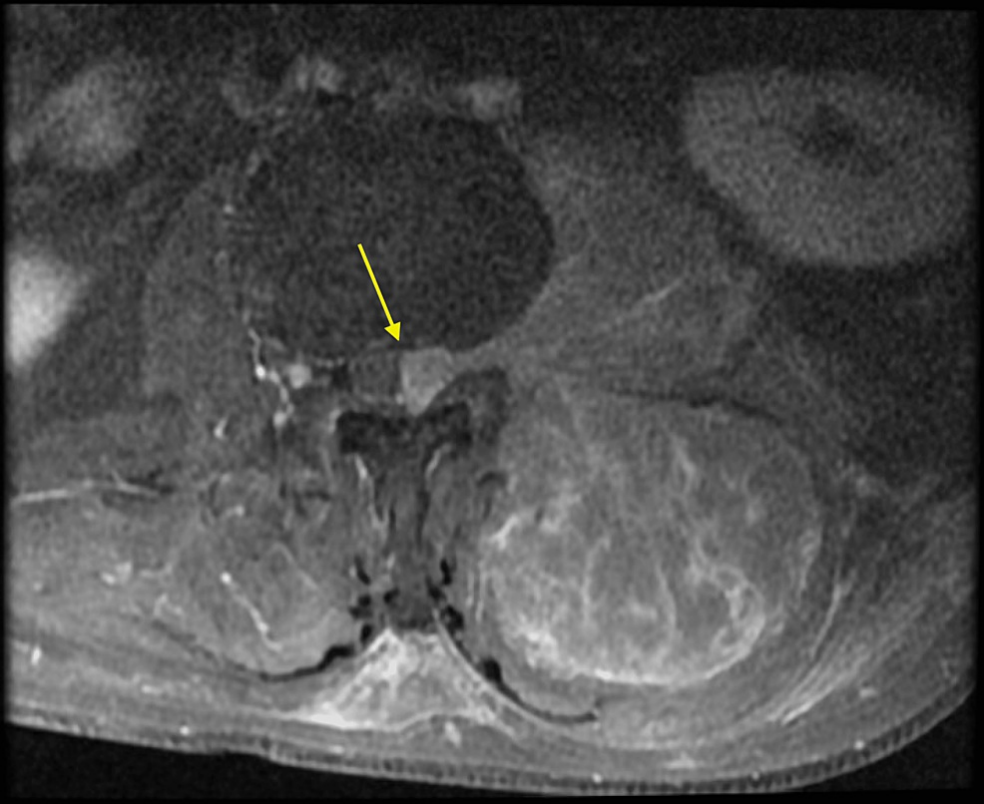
Protrusion of the soft tissue mass into the central canal shown by the T1-weighted axial view with contrast
